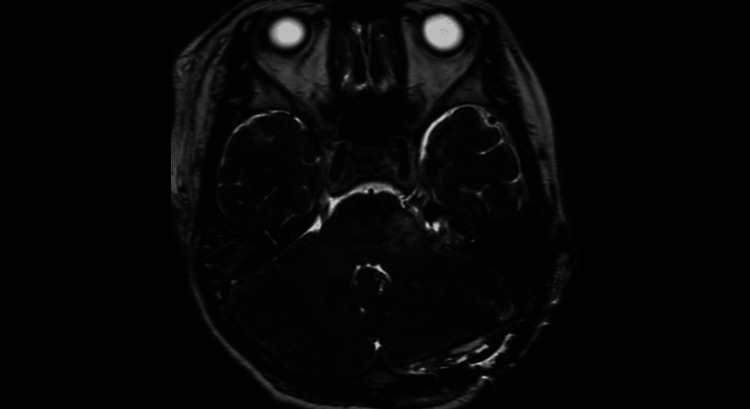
Immediately after reoperation, MRI T2-weighted image The tumor had been completely removed, and the compression on the brainstem had been relieved.
